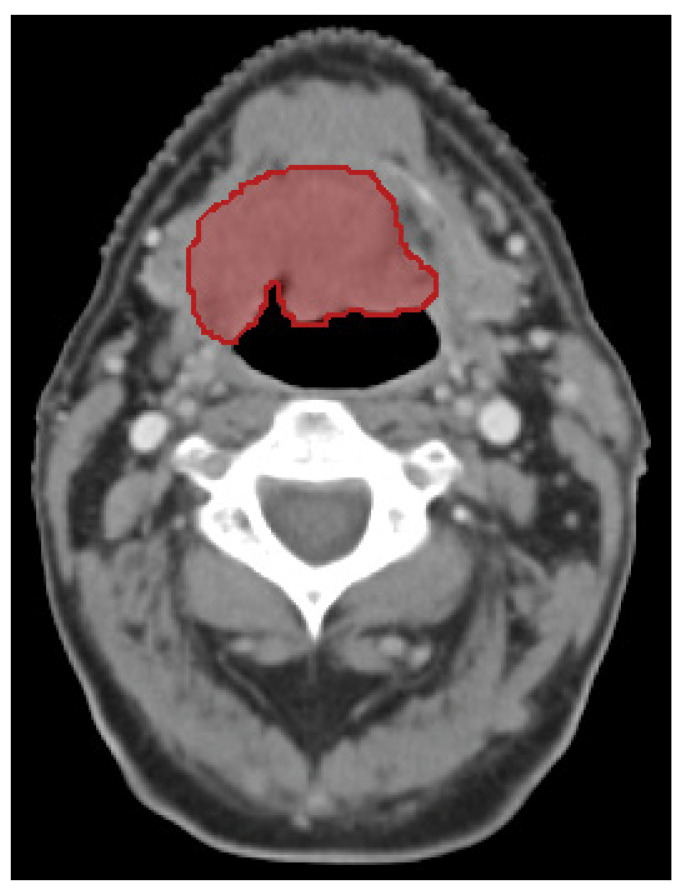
Computed tomography image of patient with stage IVA oropharyngeal cancer in transverse plane. The primary tumour is shown outlined in red.
